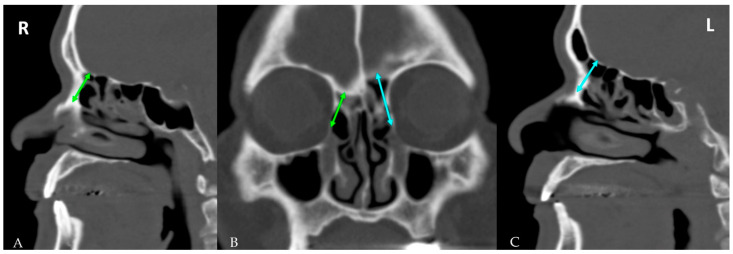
The closest distance between the lacrimal sac and anterior skull base in a parasagittal view (A,C) and coronal view (B) on computed tomography. This distance was shorter in the right aplastic frontal sinus (A) than in the left hypoplastic frontal sinus (C).
